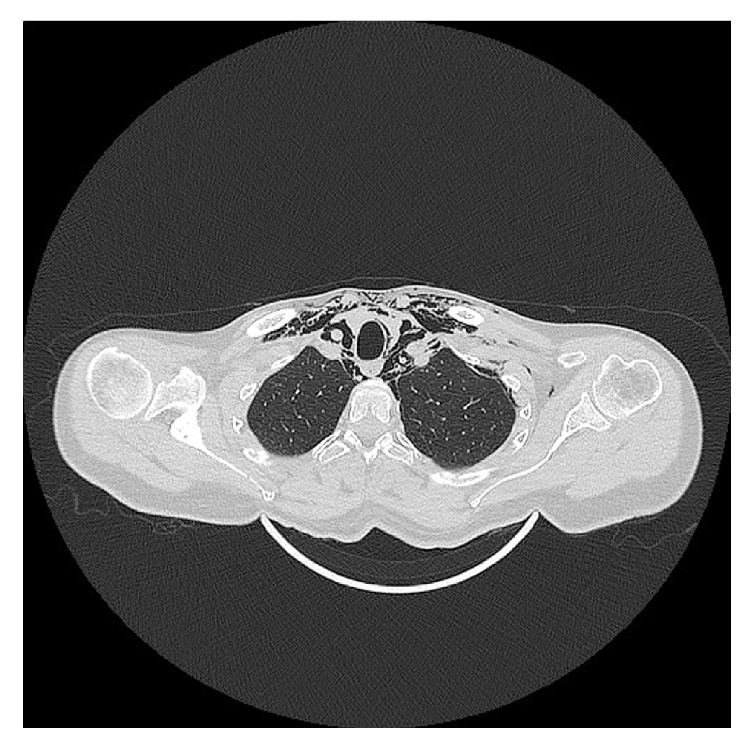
Computed tomographic axial view of the emphysema in the upper thorax, splaying the vascular bundle and running posterior to the clavicles with widening of the pectoral muscles.
